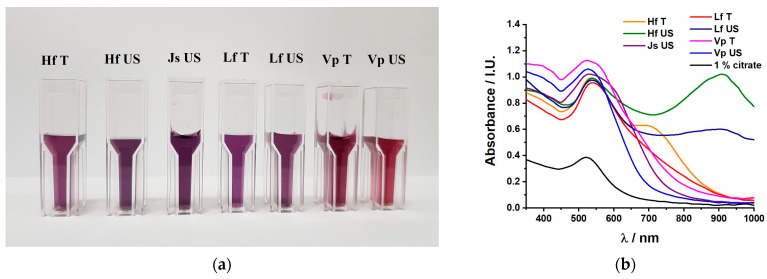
B-AuNP colloidal solutions of pressure enhanced (T) and ultrasound-assisted (US) sea buckthorn extracts (HfT and HfUS); lavender extracts (LfT and LfUS); grape extracts (VpT and VpUS) and ultrasound-assisted walnut extract (JsUS): (a) Picture of the as-prepared b-AuNP colloidal solutions; (b) UV–Vis absorption spectra of citrate-reduced AuNP (1% citrate) and b-AuNPs from (a).
